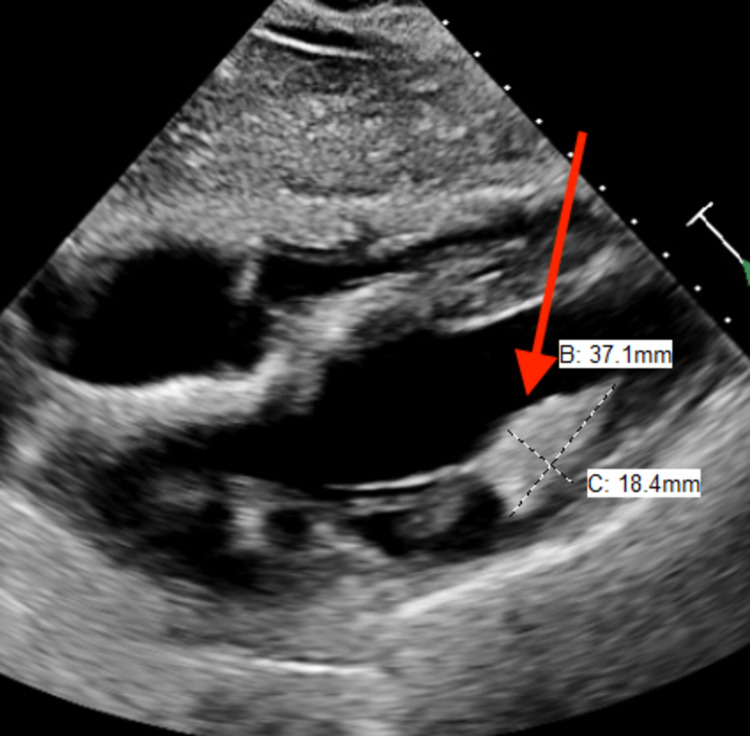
Parasternal long axis view showing rhabdomyoma arising from lateral wall of left ventricle and protruding into the left ventricular cavity
